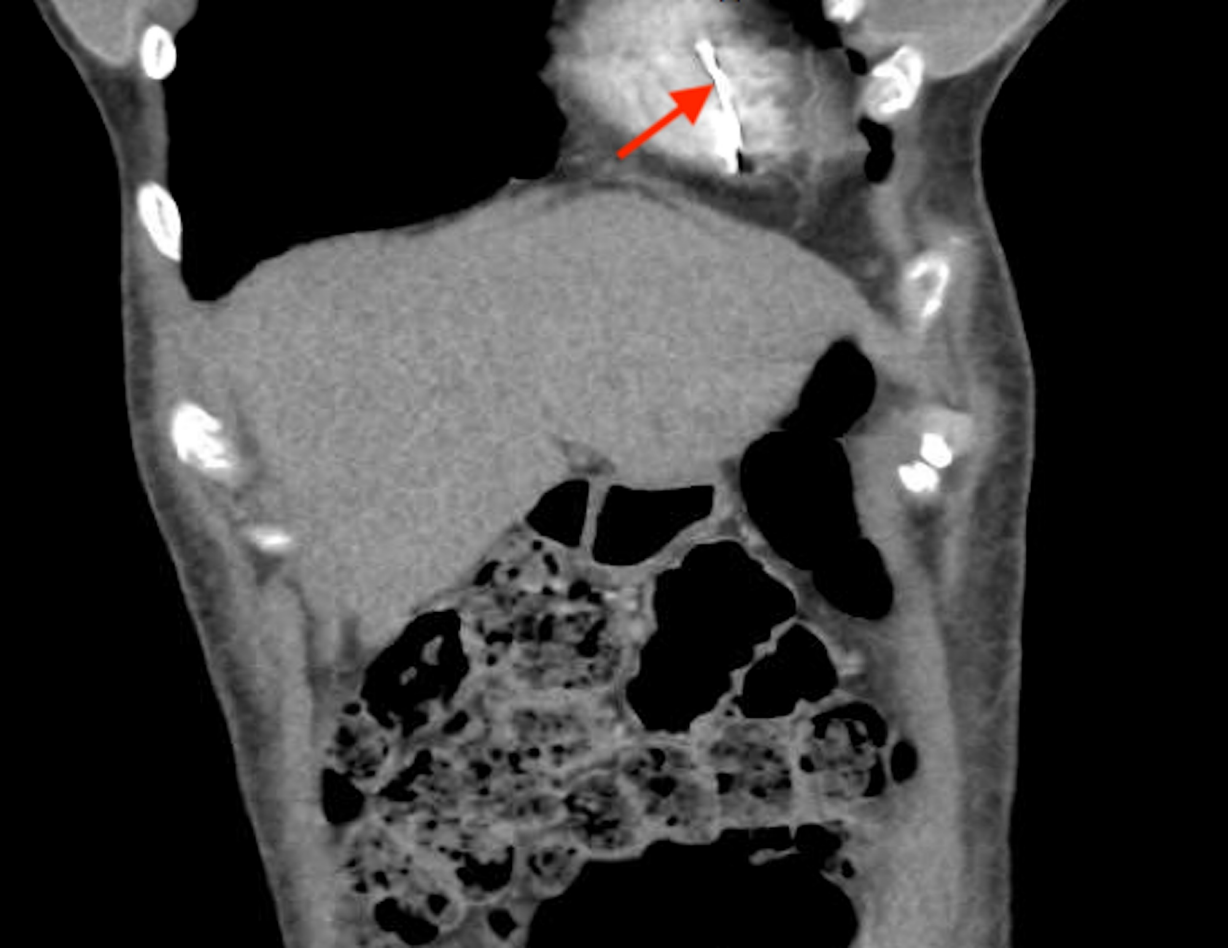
CT of the chest and abdomen (coronal section) showing the migrated inferior vena cava filter strut (red arrow).
